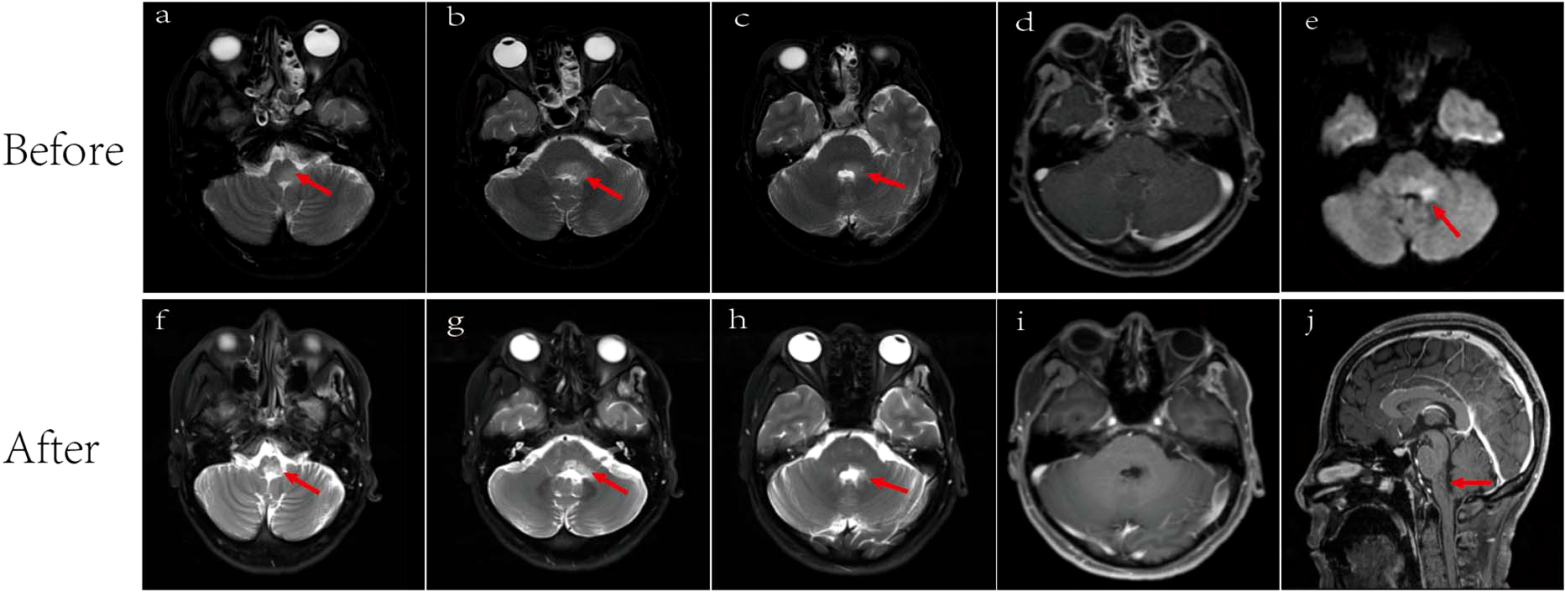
Brain MRI. Lesions (arrowed) on brain MRI images before (A–E) and after (F-J) antiviral and immunotherapy showing obviously manifested shrinkage after treatment. (A) T2-weighted showed medulla oblongata lesion. Lesion area: 2.4 cm^2^; (B) T2-weighted showed dorsal aspect of the brainstem and left cerebellar peduncle lesions. Lesion area: 3.3 cm^2^; (C) T2-weighted showed dorsal aspect of the brainstem and left cerebellar peduncle lesions. Lesion area: 1.8cm^2;^
